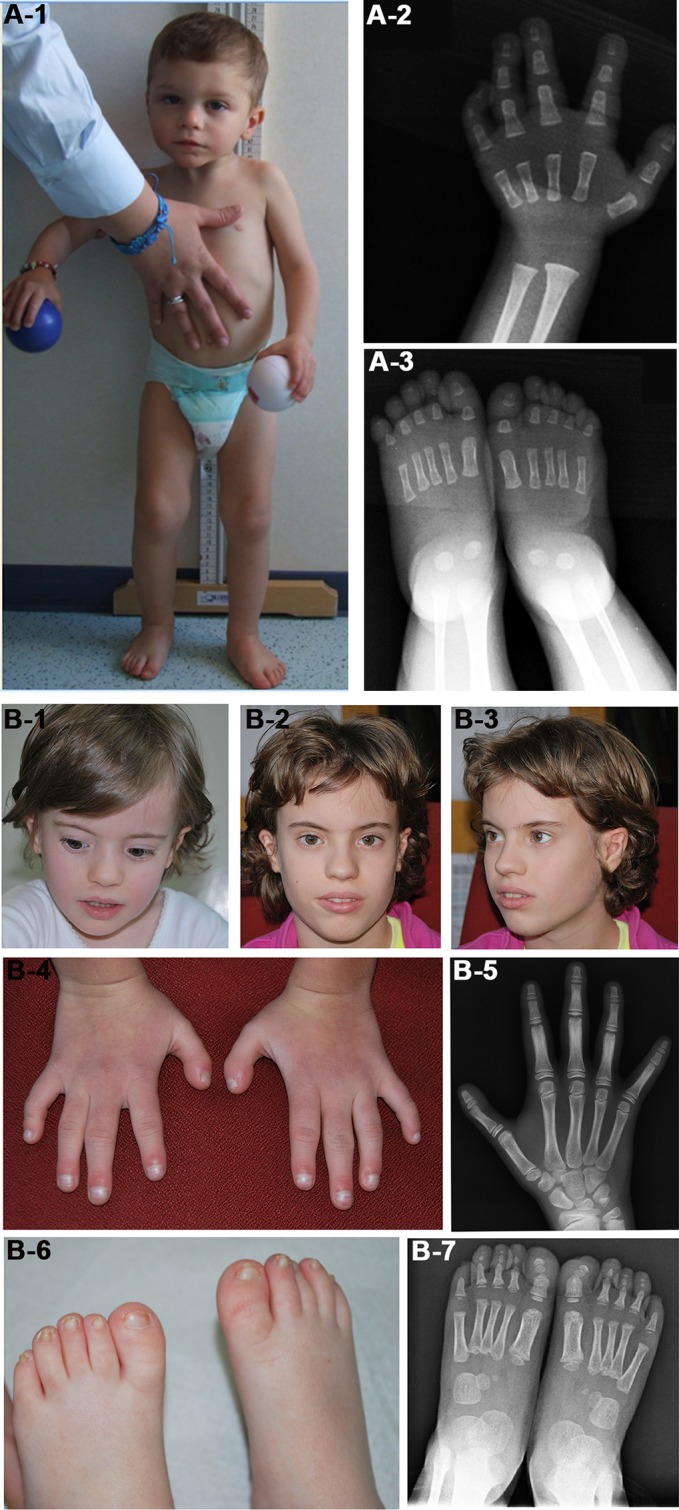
Photographs of patients 7 (A) and 20 (B). (A-1) Face and whole body of patient 7 at 28 months showing proportionate short stature, mild craniofacial dysmorphisms, that is, triangular face and slight micrognathia, and flat feet. (A-2 and 3) Left hand and feet X-rays of the patient at 11 months showing (A-2) absence of the carpal bone nuclei, suggestive of a delayed bone age, fifth finger clinodactyly with hypoplastic and triangular shaped intermediate phalanx and distal phalanx hypoplasia, and (A-3) short first toes. The absence of bone nuclei at the carpus (A-2) and their presence at the tarsus (A-3) suggest a non-uniform pattern of skeletal maturation. (B-1, B-2, and B-3) Facial appearance of patient 20 at 4 years (B-1) and 10 years (B-2, B-3) showing prominent forehead and triangular face more evident in childhood. (B-4) Small hands with proximal placement and ulnar side bowing of first fingers, and clinodactyly of fifth fingers. (B-5) Left hand X-rays of the patient at 10 years showing hypoplasia of distal phalanges. (B-6) Small feet with short and broad halluces, right over-riding toes, and small/deep-set nails. (B-7) Feet X-rays of the patient at 4 years showing proximal placement of fifth metatarsals, absence of distal phalanges in second and fifth toes, absence of middle phalanges in fifth toes, and phalanges hypoplasia.
